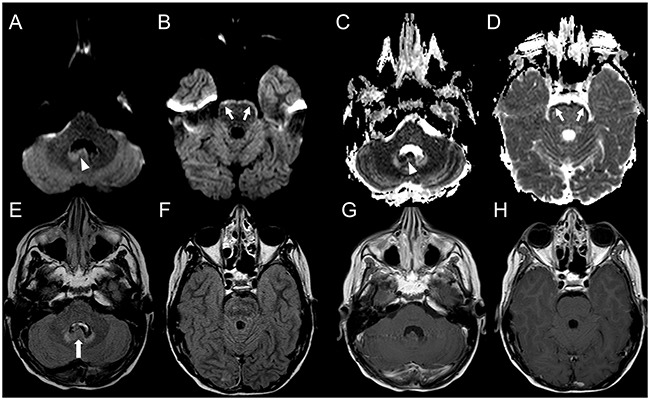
Brain MRI findings in a 12-year-old boy with CNS ET, NOS relapse and linear leptomeningeal involvement visible only on DWI DWI (A, B) and corresponding ADC (C, D) maps show two adjacent focal nodular ependymal relapsing lesions with decreased diffusivity located in the right posterolateral recess of the IV ventricle and along the nodule of vermis (arrowheads, A, C). There is also linear decreased diffusivity along the ventral margin of the brainstem in keeping with linear leptomeningeal involvement (short thin arrows, B, D). FLAIR images (E, F) show a reduced representation of the right posterolateral recess of the IV ventricle and focal prominence of the nodule of vermis (thick arrow, E). Post-contrast T1-weighted images (G, H) do not reveal enhancing lesions.
